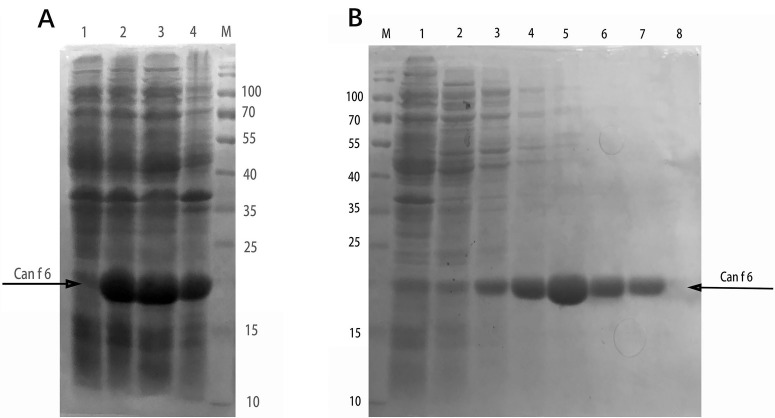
Expression and purification of Can f 6 in *E. coli* A. Lane M, protein molecular weight standard; Lane 1, non-induced recombinant Can f 6 whole cell lysate; Lane 2, IPTG-induced recombinant Can f 6 whole cell lysate; Lane 3, supernatant fraction after ultrasonication; Lane 4, precipitation fraction (inclusion bodies) after ultrasonication. The Can f 6 was denoted with an arrow; B. SDS-PAGE after affinity chromatography of Can f 6. Lane M, protein molecular weight standard; Lane 1, Unbinding fractions of the supernatant of Can f 6; Lane 2, washing with 10 mM imidazole; Lane 3, washing with 50 mM imidazole; Lane 4, washing with 100 mM imidazole; Lane 5, washing with 150 mM imidazole; Lane 6, washing with 200 mM imidazole; Lane 7, washing with 250 mM imidazole; The arrow point to the purified Can f 6.
